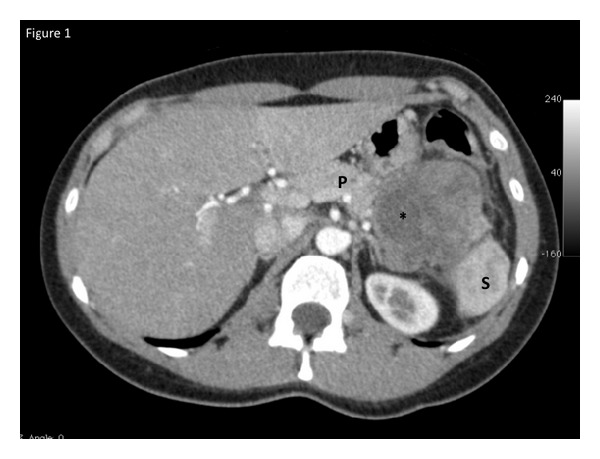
Contrast enhanced CT scan of the abdomen revealing the solid pseudopapillary tumor in the pancreatic tail (P). The spleen (S) is visible distally and an area of cystic degeneration is noted centrally marked by an asterix.
